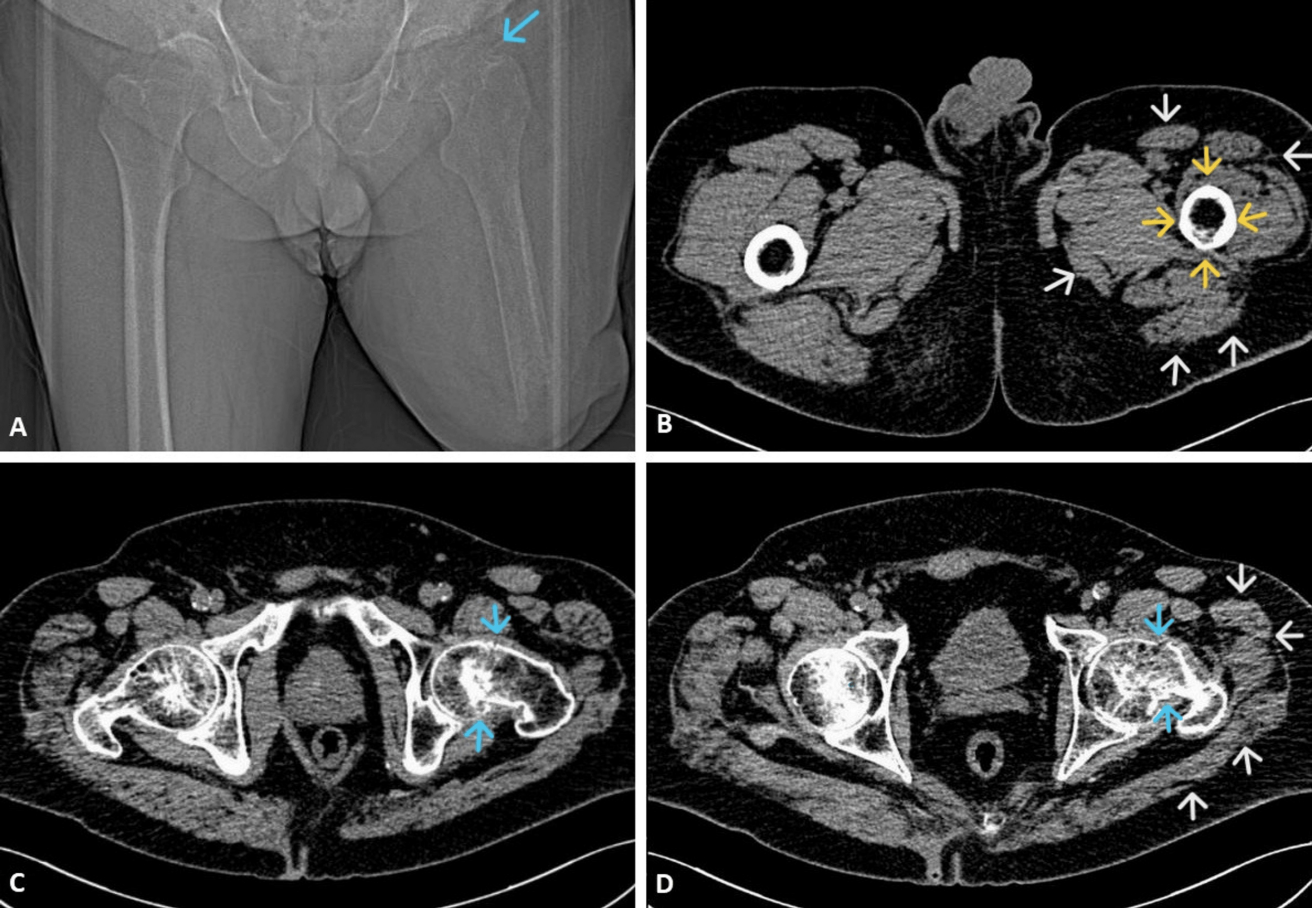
Preoperative CT imaging. A - coronal scout view demonstrating displaced subcapital femoral neck fracture of the left hip; B - axial CT slice showing cortical thinning of the femoral shaft (yellow arrows) and marked muscle atrophy (white arrows); C - axial CT slice highlighting the fracture line (blue arrows); D - axial CT slice showing both muscle atrophy (white arrows) and the fracture line (blue arrows).
